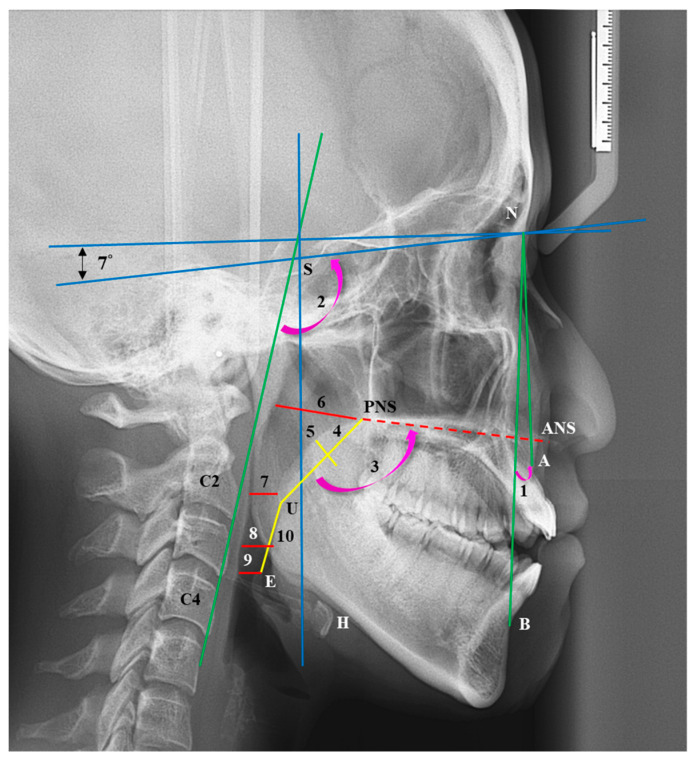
Cephalometric landmarks. S: sella; N: nasion; A point; B point; ANS: anterior nasal spine; PNS: posterior nasal spine; H: hyoid bone; C2: The most anterior inferior point of the second cervical vertebra; C4: The most anterior inferior point of the fourth cervical vertebra; U: uvula; E: epiglottis. Reference lines: The *X* axis in the X-rays was set as 7° upward of the line connecting the S and N, using N as the fulcrum. The line passing through S perpendicular to the *X* axis was considered to be the *Y* axis. Angular and linear measurements. 1: ANB angle; 2. C2C4-SN angle; 3: Palatal angle; 4: SPL (soft palate length); 5: SPW (soft palate width); 6: NPS (nasal pharyngeal airway space); 7: SPS (soft palate pharyngeal airway space); 8: TPS (Tongue pharyngeal airway space); 9: EPS (epiglottis pharyngeal airway space); 10: UE distance.
